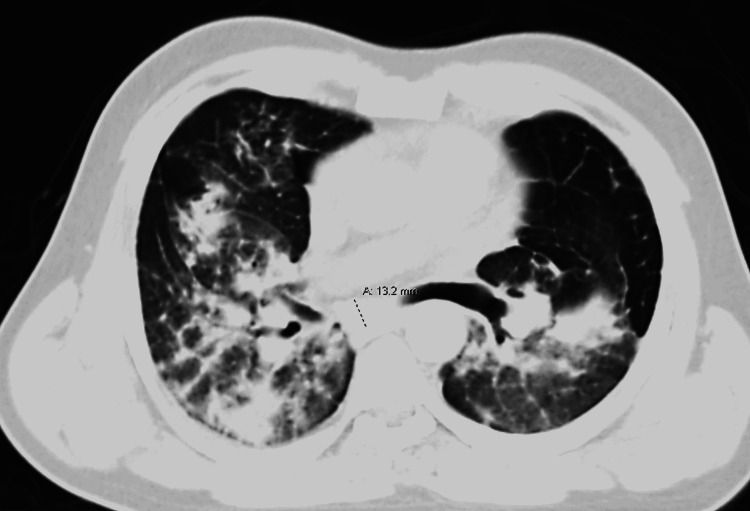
Chest CT without (w/o) contrast in the context of silicosis.
